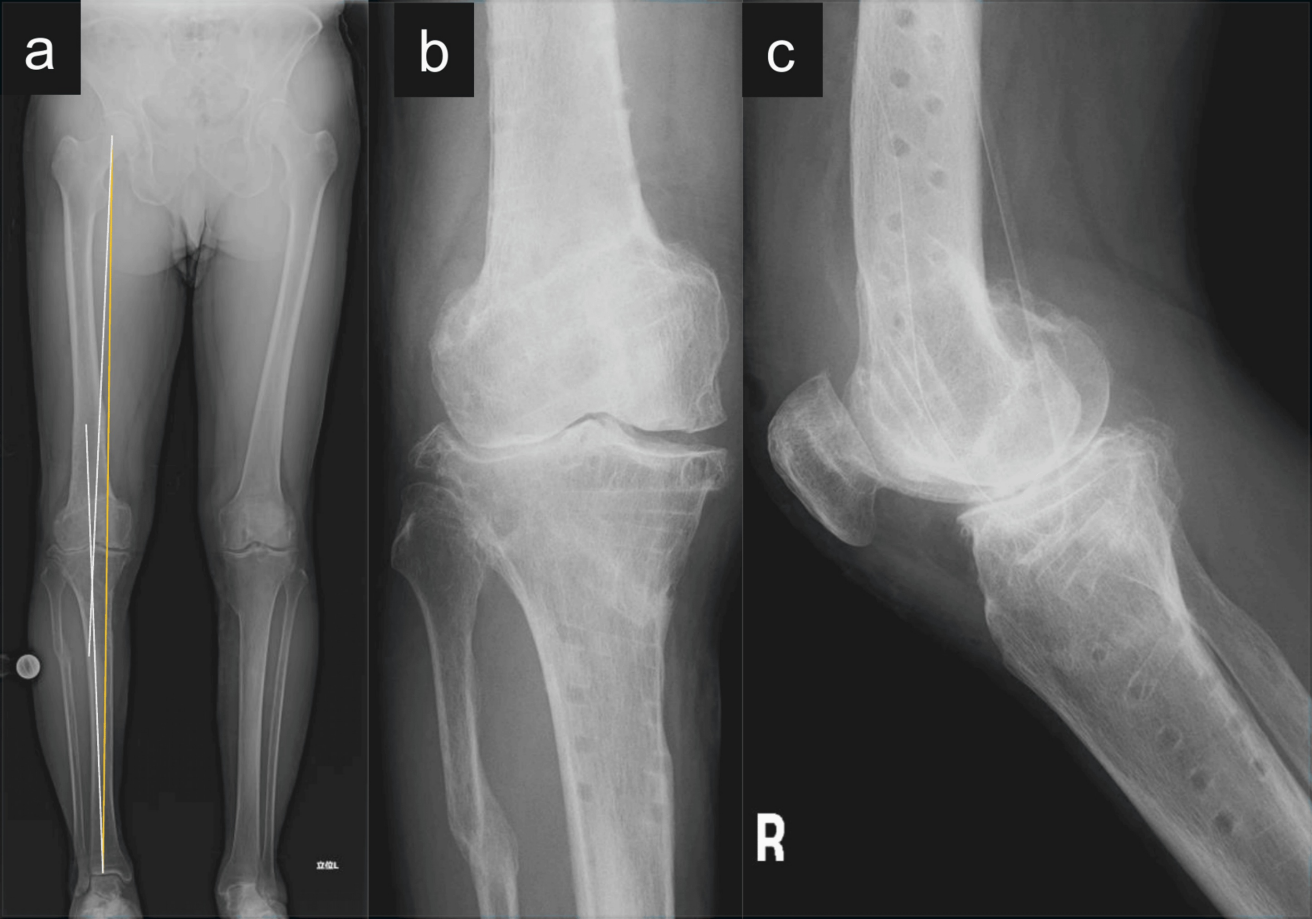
One-year postoperative radiographs of the right knee following double-level osteotomy. (a) Standing full-length anteroposterior (AP) radiograph showing maintained correction with an hip–knee–ankle angle of 6°, indicated by white lines. The mechanical axis, shown in yellow, passes through the medial 30% of the tibial plateau. (b) AP radiograph of the right knee demonstrating complete bone union at the femoral and tibial osteotomy sites.
